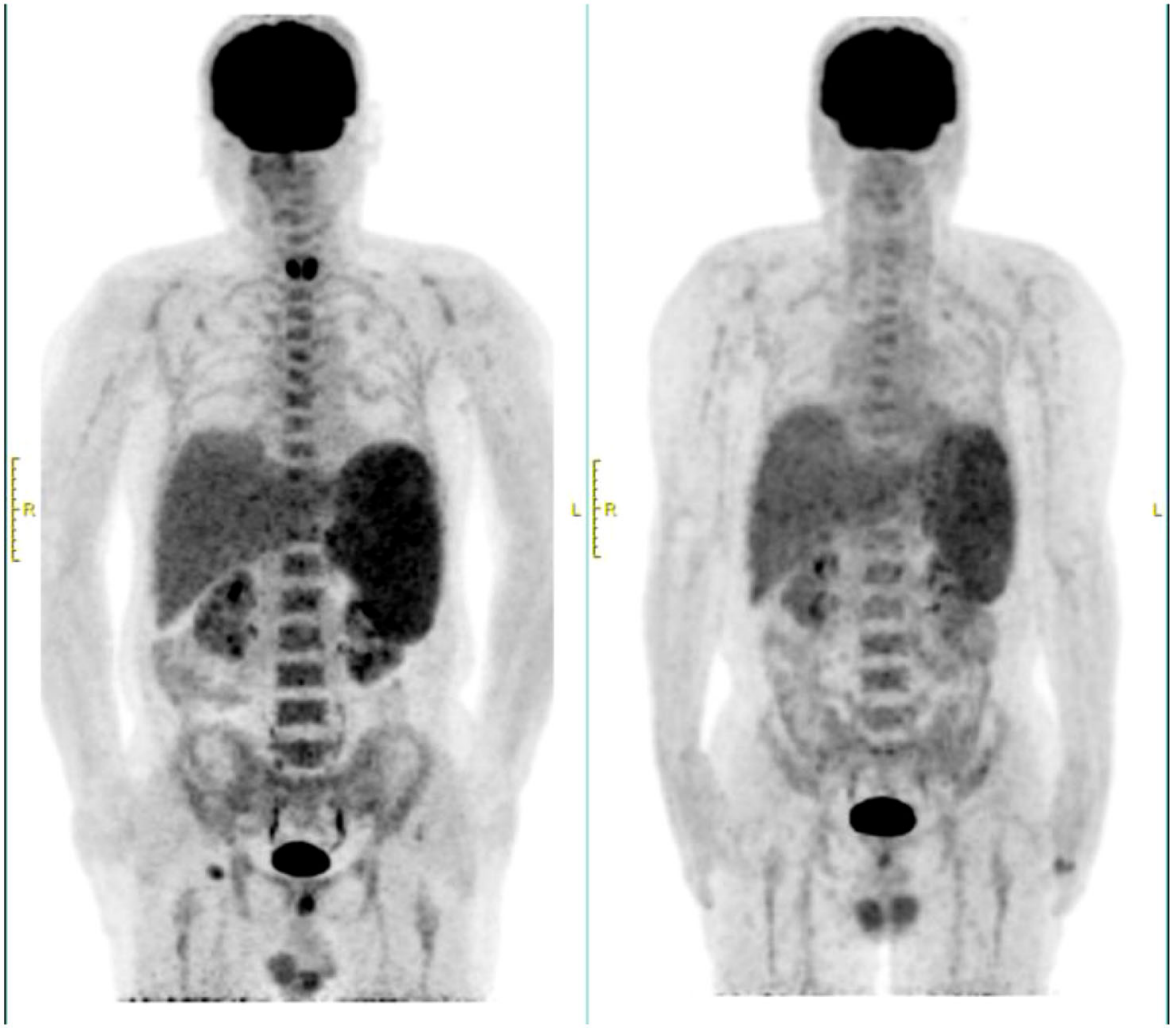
PEC/CT at recurrence and initial diagnosis. The left side was at recurrence in March 2024, and the right side was at initial diagnosis in August 2023.
